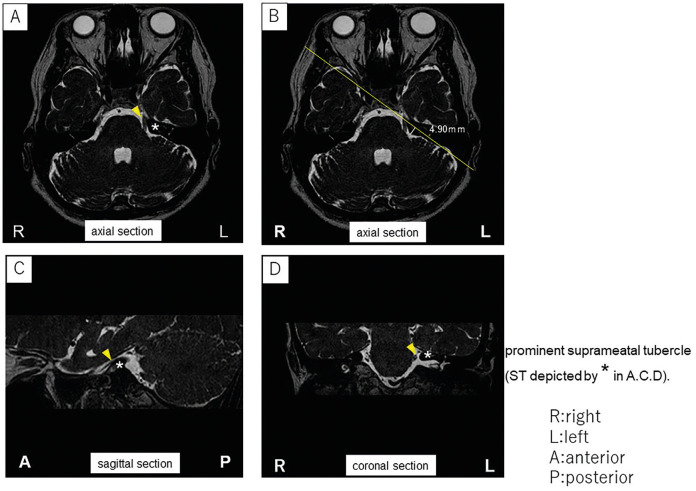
In the cerebellopontine angle area, a prominent suprameatal tubercle (ST) was observed, depicted by * in T1-weighted, contrast-enhanced axial (A), sagittal (C), and coronal (D) views, with contact noted between the superior cerebellar artery and the trigeminal nerve root (yellow arrows). B: The height of ST on the MRI was 4.90 mm.
